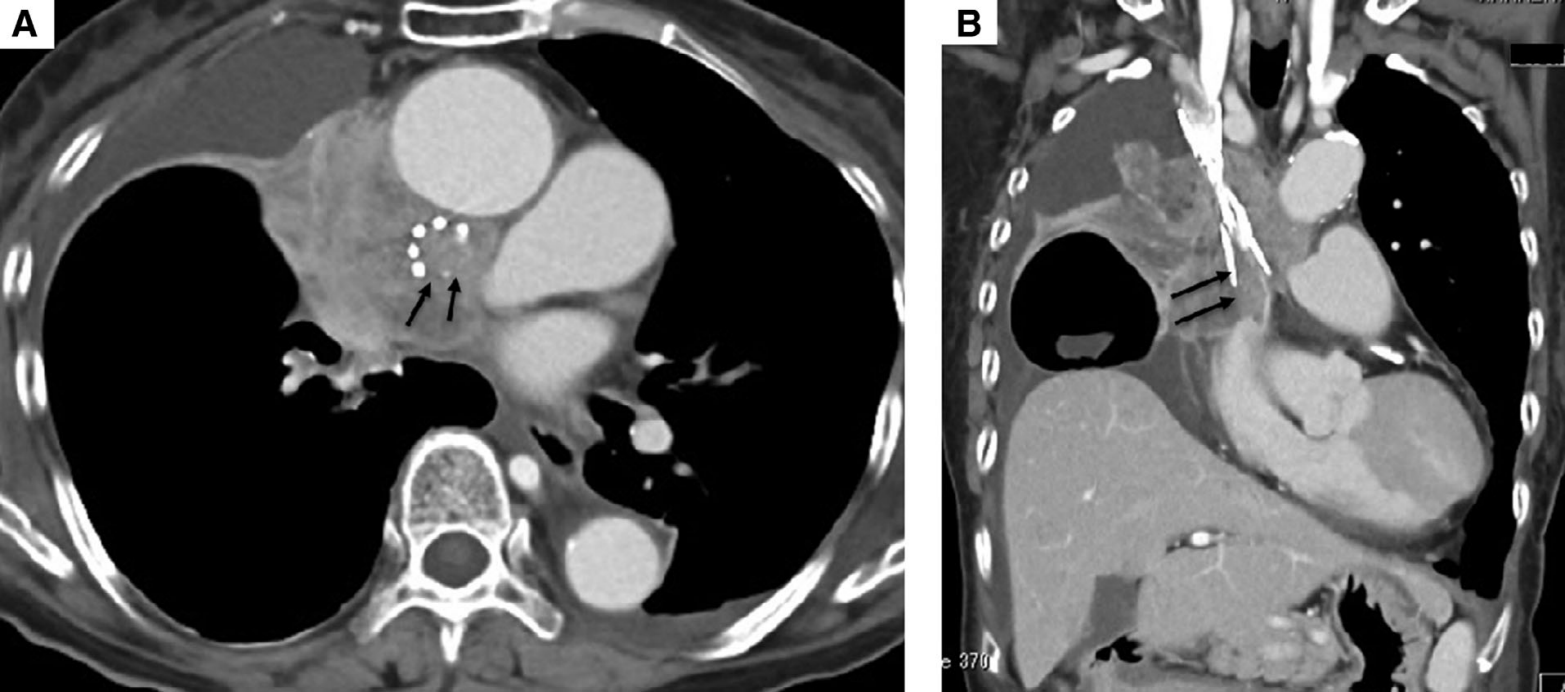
Contrast‐enhanced computed tomography (CT) images of the thorax in the axial (A) and coronal (B) planes. Chest CT showed proximal obstruction of the initial superior vena cava (SVC) stent by tumour ingrowth (arrows).
